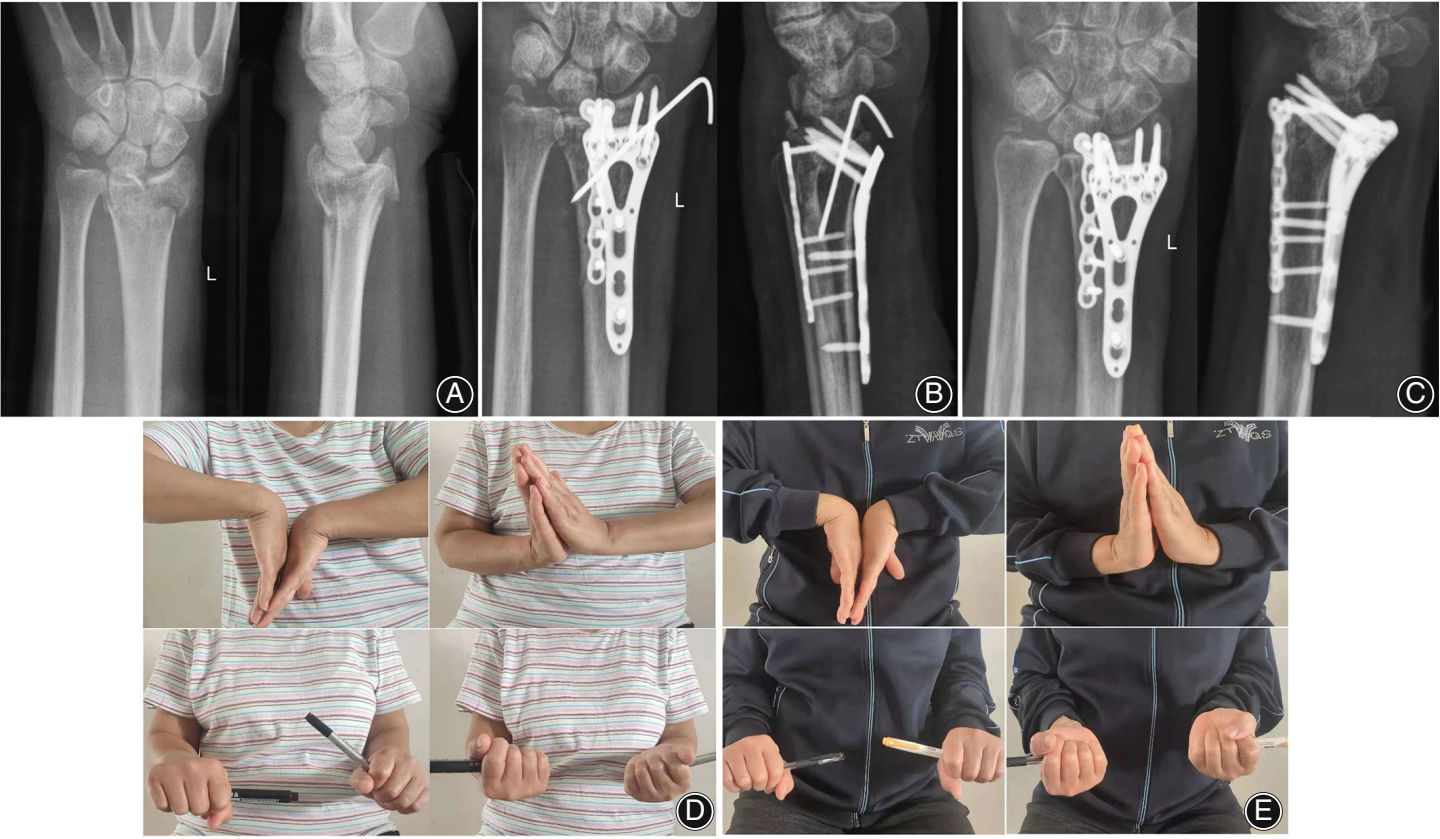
A 70‐year‐old woman suffered from malunion of distal radius fractures, radius shortening, and the loss of volar tilt. (A) Anteroposterior and lateral radiographs of the left radiocarpal joint. (B) Anteroposterior and lateral radiographs of the left radiocarpal joint at 3 months show restoration of the radius height and volar tilt. (C) Anteroposterior and lateral radiographs of the radius length and volar tilt within the normal range at 18 months. (D) Wrist function was evaluated by flexion and extension of wrist and pronation and supination of the forearm at 3 and (E) 18 months postoperatively
